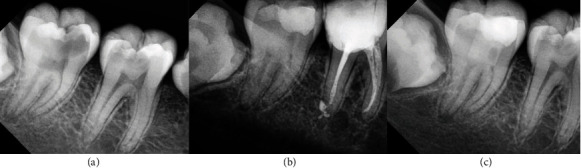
Permanent tooth 47. The image (a) shows diagnostic X-ray, image (b) immediate postoperative, and image (c) control at 32 months.
